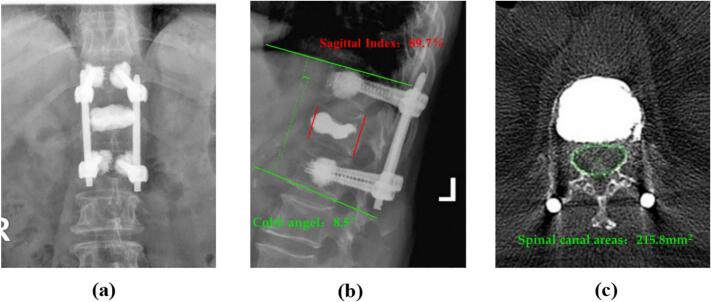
Anteroposterior and lateral X-ray and transverse CT were performed 1 year after operation.
